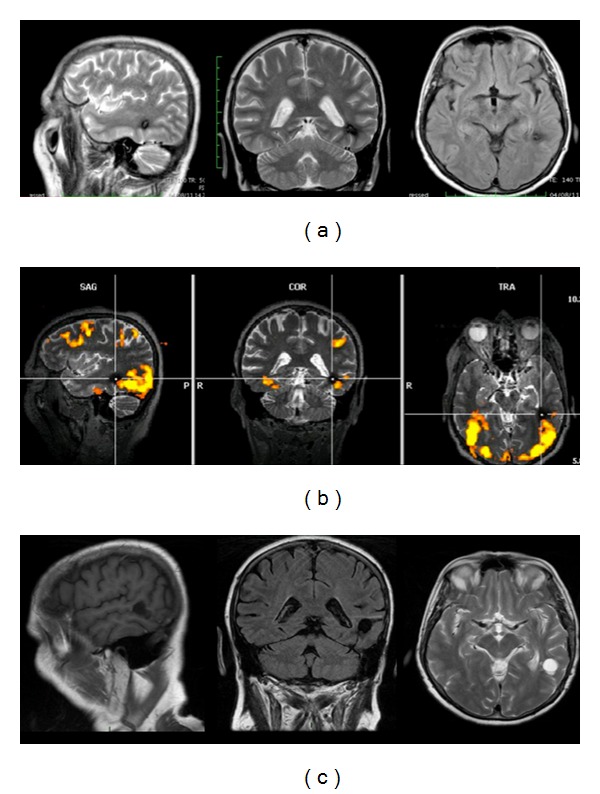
(a) Preoperative MRI sequences (sagittal T2, coronal T2, and axial Flair) showing a 1 cm mixed-intensity lesion with a hypointense rim into the subcortical region of the middle temporalgyrus (MTG) directly in contact to the stems and/or terminations of the subcortical language pathways of this region (i.e., inferior fronto-occipital fasciculus, arcuate fasciculus, and indirect posterior component of superior longitudinal fascicle). (b) The preoperative 4T f-MRI (MR Scanner MedSpec, Bruker) showing the main activation during language task of the posterior temporal lobe on sagittal, coronal, and axial planes. The f-MRI demonstrated significant activations at the level of the posterior thirds of inferior temporal gyrus (ITG) and MTG in the BA 37 and in the occipital laterobasal cortex in the BA 19. No activations were detected at the level of the classical Wernicke's area at the level of the posterior thirds of the MTG and superior temporal gyrus (STG) and perisuperior temporal sulcus (STS) in the BA 22. (c) The postoperative MR sequences (sagittal T1, coronal Flair, and axial T2) demonstrated the complete resection of the cavernous angiomas (CA) and of the perilesional hemosiderin gliotic rim. CA: cavernous angiomas; ITG: inferior temporal gyrus; MTG: middle temporal gyrus; STG: superior temporal gyrus; STS: superior temporal sulcus.
